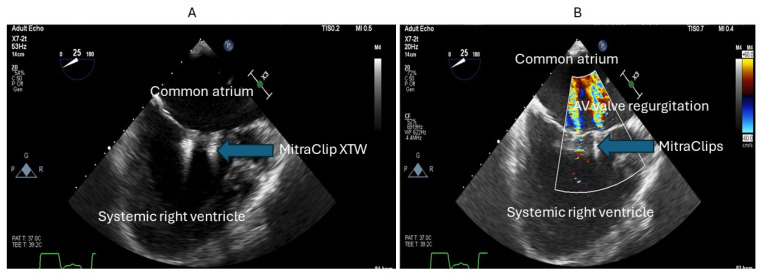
Transesophageal echocardiogram demonstrating two MitraClips XTW (A), and an improvement in atrioventricular (AV) regurgitation severity (B).
